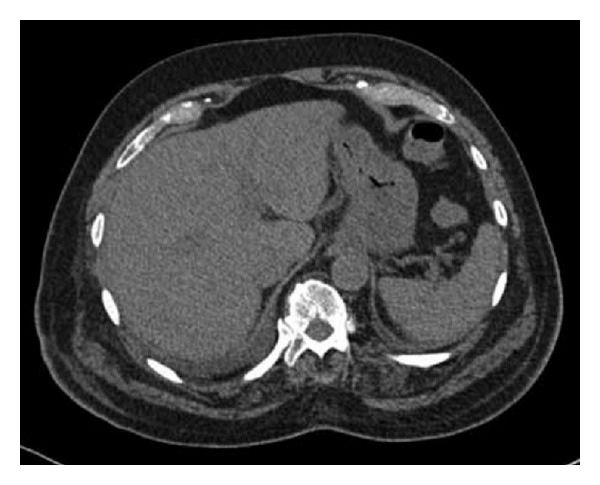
CT showing resolution of the hepatic portal venous gas (Case 1).
